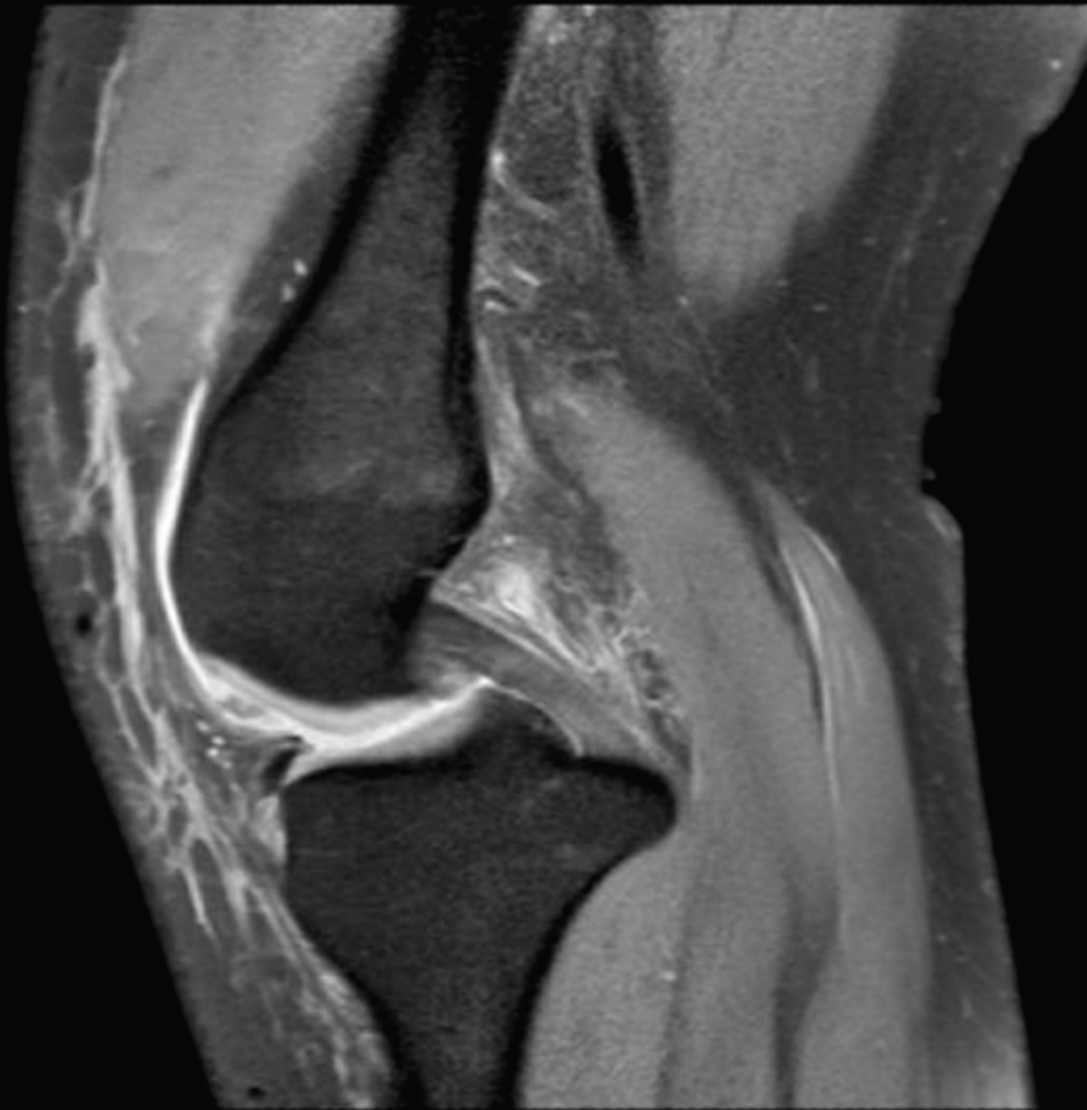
Sagittal magnetic resonance image of right knee.
